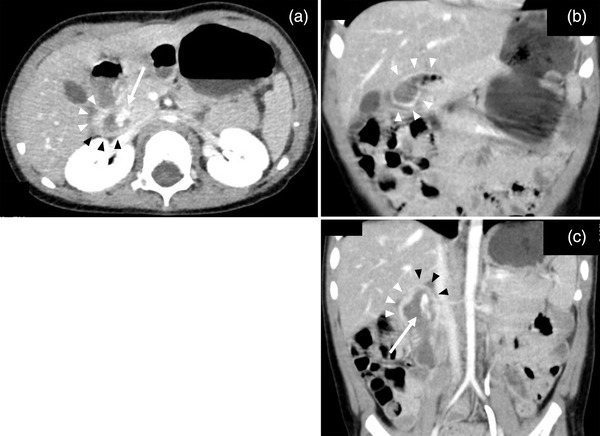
Abdominal contrast‐enhanced computed tomography showing extravasation in the descending part of the duodenum, and the mildly thickened and unevenly enhanced wall of the bulb to the descending part. (a) Axial view. (b, c) Coronal view. Arrows indicate contrast extravasation. Black arrowheads indicate the thickened and weakly enhanced wall and white arrowheads indicate the thickened and strongly enhanced wall
